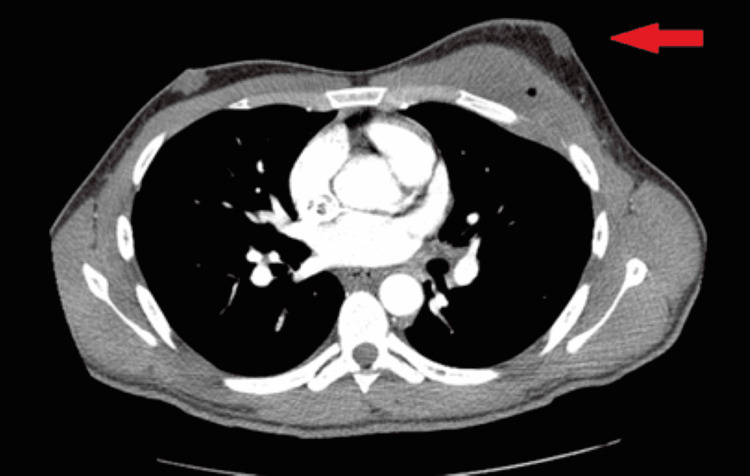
Computed tomography angiography of the chest Phlegmon-like subcutaneous lesion with skin thickening and expansile erosion of the anterior aspect of the left fourth rib.
